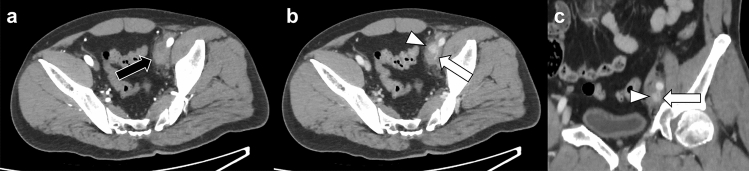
Venous injury due to a traffic accident in a 50 year-old man. a Arterial phase pelvic CT scan shows no extravasation of contrast material within the hematoma (black arrow). b, c Venous phase CT scan (b: axial view, c: coronal view) shows extravasation of contrast material (arrowheads) with a narrowed external iliac vein (arrows). CT, computed tomography
